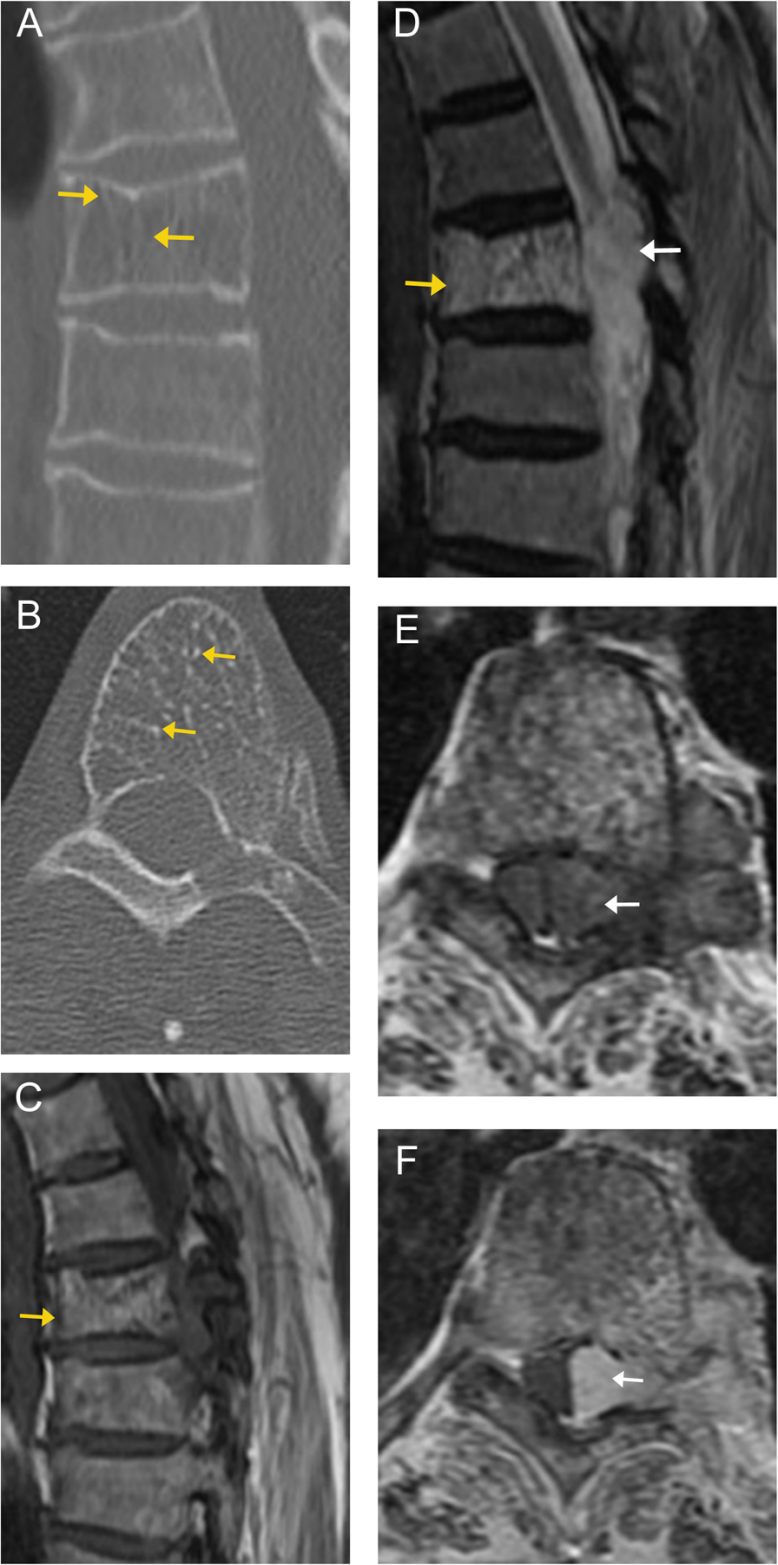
Compressive vertebral venous vascular malformation. Sagittal (a) and axial (b) CT images in the bone window of the thoracic spine demonstrate decreased attenuation of the T4 vertebral body, as well as the left pedicle and transverse process. A characteristic “polka-dot” sign is seen in the vertebral body on the axial image, with high attenuation dots representing replacement of normal cancellous bone by thick, vertical trabeculae (orange arrows) surrounded by vascular lacunes and fatty marrow. Sagittal MR images demonstrate a heterogenous, predominantly high signal lesion on both T1 (c) and T2 (d) weighted sequences involving the T4 vertebral body (orange arrows), with lobular extension into the spinal canal (white arrows). Axial T1 pre- (e) and post-contrast (f) images demonstrate avid enhancement of the lesion. Again, there is extension of the lesion from the vertebral body into the spinal canal, with resulting compression of the spinal cord
